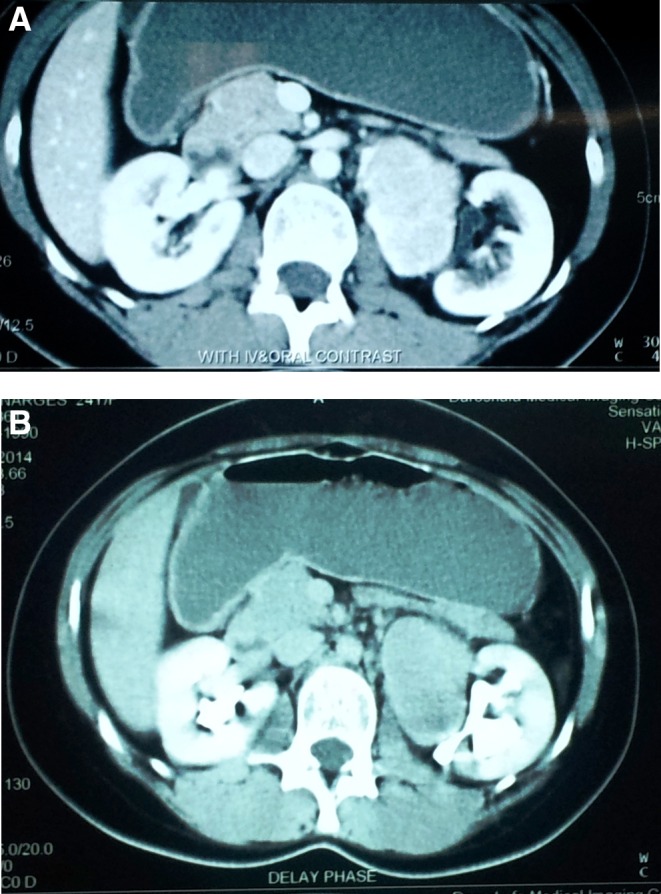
CT scan imaging revealed a mass in the left renal hilum that enhanced after injection of the intravenous contrast (A). The lesion did not invade the pyelocalyceal system (B).
